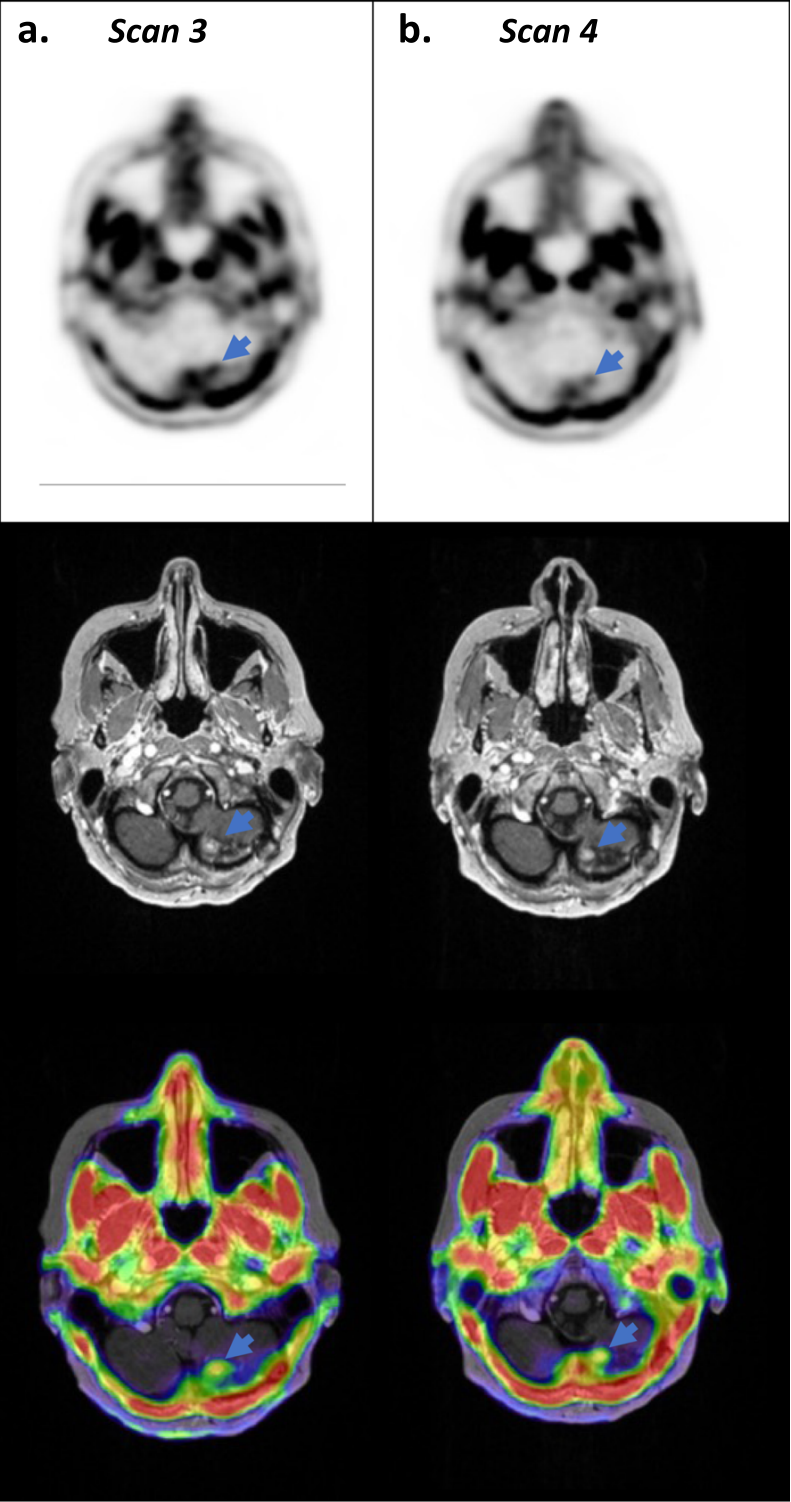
Static PET, T1 MRI_CE_ and fused PET/MR images of the cerebellar tumour in scans 3 and 4. Axial view of the patient’s left hemisphere caudomedial cerebellar metastasis (arrow) acquired using hybrid PET/MR imaging at time points 3 and 4. Static PET (20–35 min), T1 MRI_CE_ and fused PET/MR images are shown from the top to bottom rows, respectively. Columns a and b show scans 3 and 4. The SUV scale for static cerebellar PET images was 0–2, and the SUV scale of fused PET/MR images was 0.45–2
